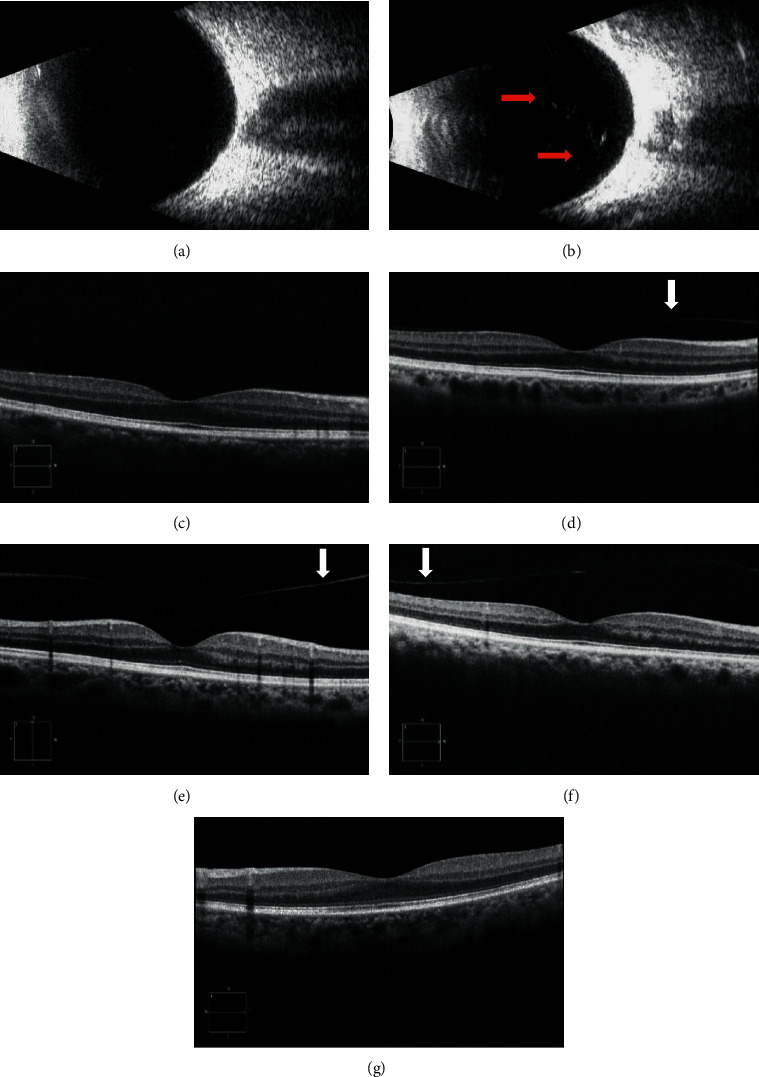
Posterior vitreous detachment (PVD) grading based on B-scan ultrasonography (US) and optical coherence tomography (OCT). (a) No PVD, B-scan US showing the absence of a hyperechoic membrane over the retinal pigment epithelium (RPE) layer. (b) Complete PVD, presence of a hyperechoic membrane (red arrows) in addition to the posterior RPE layer and completely detached from the RPE layer. (c) Stage 0, no PVD. (d) Stage 1, focal perifoveal PVD involving one to three quadrants. (e) Stage 2, perifoveal PVD across all quadrants, with persistent attachment to the fovea. (f) Stage 3, detachment of the posterior vitreous face from the fovea, with persistent attachment to the optic disc. (g) Stage 4, complete detachment of the posterior vitreous cortex (PVC) from the retina and optic disc, the PVC is not observed. White arrows denote the detached PVC.
